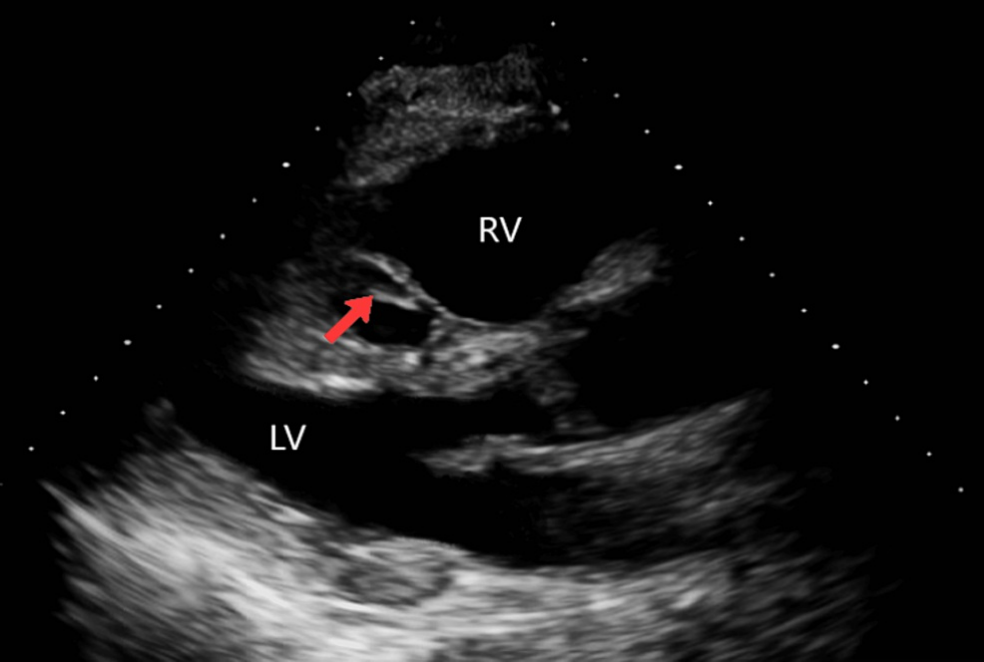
Parasternal short axis view in transthoracic echocardiogram with red arrow pointing at a tubular structure representing the venous stent
